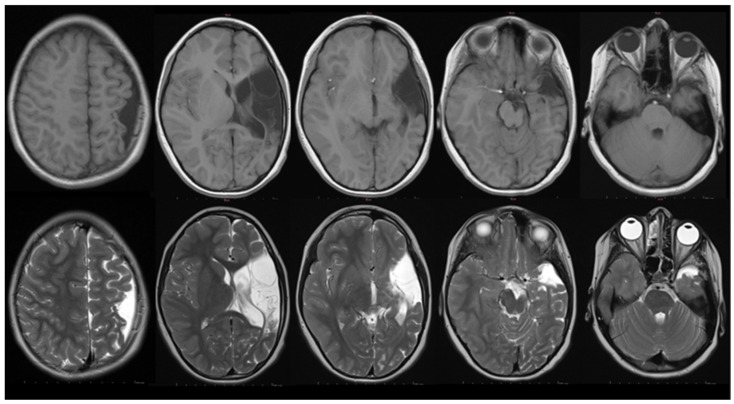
MRI scan: T1 (upper row) and T2 (lower row) weighted MRI images of the patient in the axial plane. The columns show the following axial levels: the central region (first column), basal ganglia (second column), thalami (third column), mesencephalon (fourth column), and pons (fifth column).
